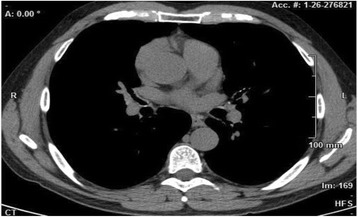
HRCT showed irregularity in main bronchi
